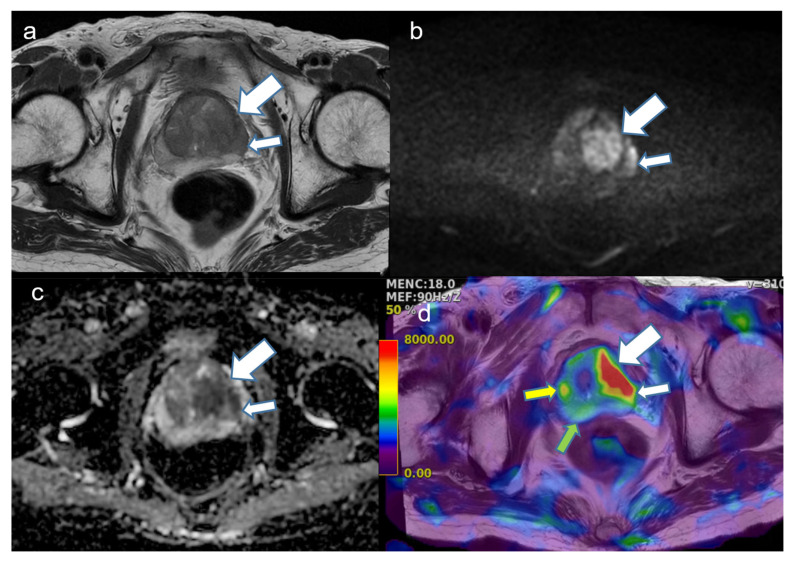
A representative case of a 72-year-old man with prostate cancer with a Gleason score of 7 (4 + 3) in the transition zone. (a) T2-weighted image shows a 3 cm low-signal-intensity (SI) lesion (large arrow) in the left transition zone (TZ) with an extension (small arrow) to the neighboring peripheral zone (PZ). It is difficult to demarcate low SI in the benign prostatic hyperplasia (BPH) nodule in the right TZ. (b) Diffusion-weighted image (b value, 2000 s/mm^2^) showing a heterogeneous high SI. (c) Corresponding apparent diffusion coefficient map also showing a reciprocal low SI in the tumor. Some diffusion-restricted areas are also seen in the BPH nodule in the right TZ. (d) Elastogram fused with T2-weighted image showing a high stiffness value (red area, white arrow) in the tumor. Compared with the stiffness value of 7.3 kilopascals in the tumor, the BPH nodule (yellow arrow) was measured at 4.1 kilopascals and normal parenchyma in the PZ (green arrow) indicated 3.2 kilopascals.
